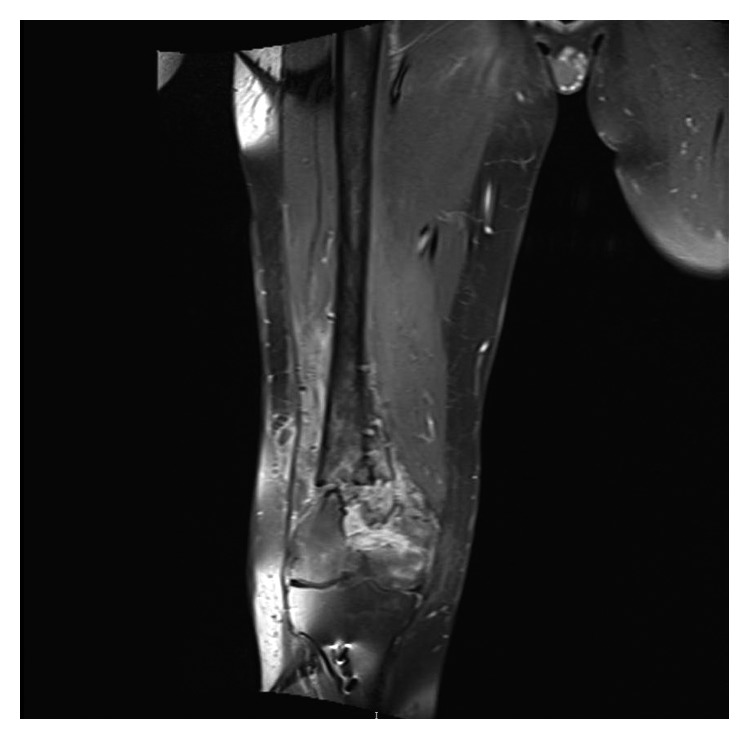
MRI of the the right knee and femur showing that the bone tissue is heavily disrupted with several infectious lytic foci in the diaphyseal and metaphyseal portion, swelling of the cancellous bone, and periosteal reaction. A cutaneous fistula draining from a fluid collection in the vastus lateralis muscle can be seen.
